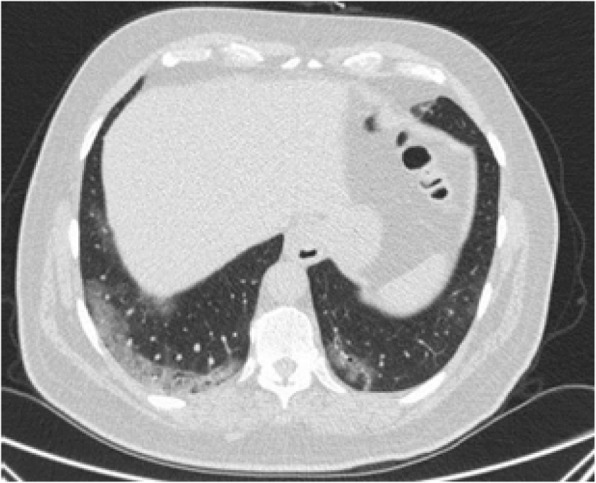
COVID-19 pneumonia: thin section CT shows bilateral subpleural GGO and septal thickening
